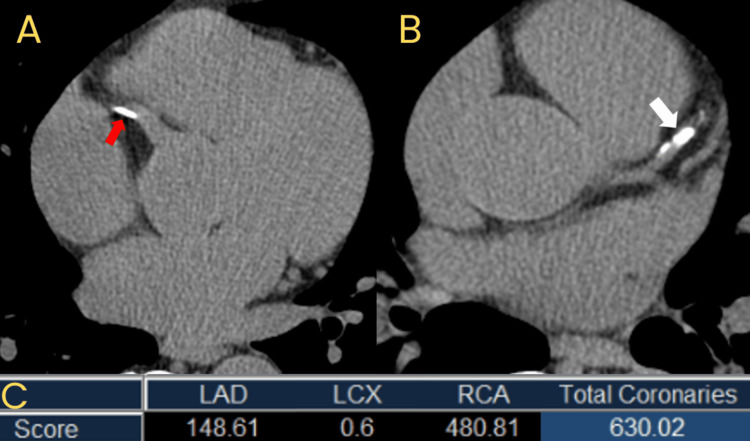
A 69-year-old male with hypercholesterolemia Axial non-contrast cardiac-gated CT images demonstrate calcified atherosclerotic plaque in the LAD (red arrow) (A) and the RCA (white arrow)(B).  Output from the calcium scoring software (C) reports the total calcium score for all calcified plaques in each vessel, and the sum total for all vessels (630 in this patient). This patient’s score falls at the 79th percentile for an age- and sex-matched cohort. CT: Computed tomography CT images are the property of Dr. Jordan Ditchek
